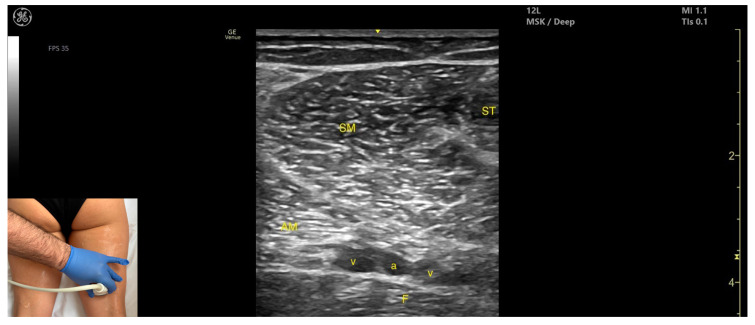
Ultrasound anatomy of *semimembranosus* (SM) with key landmarks: SM—*semimembranosus*; ST—*semitendinosus*; AM—*adductor magnus*; v—vein, a—artery, F—femur.
